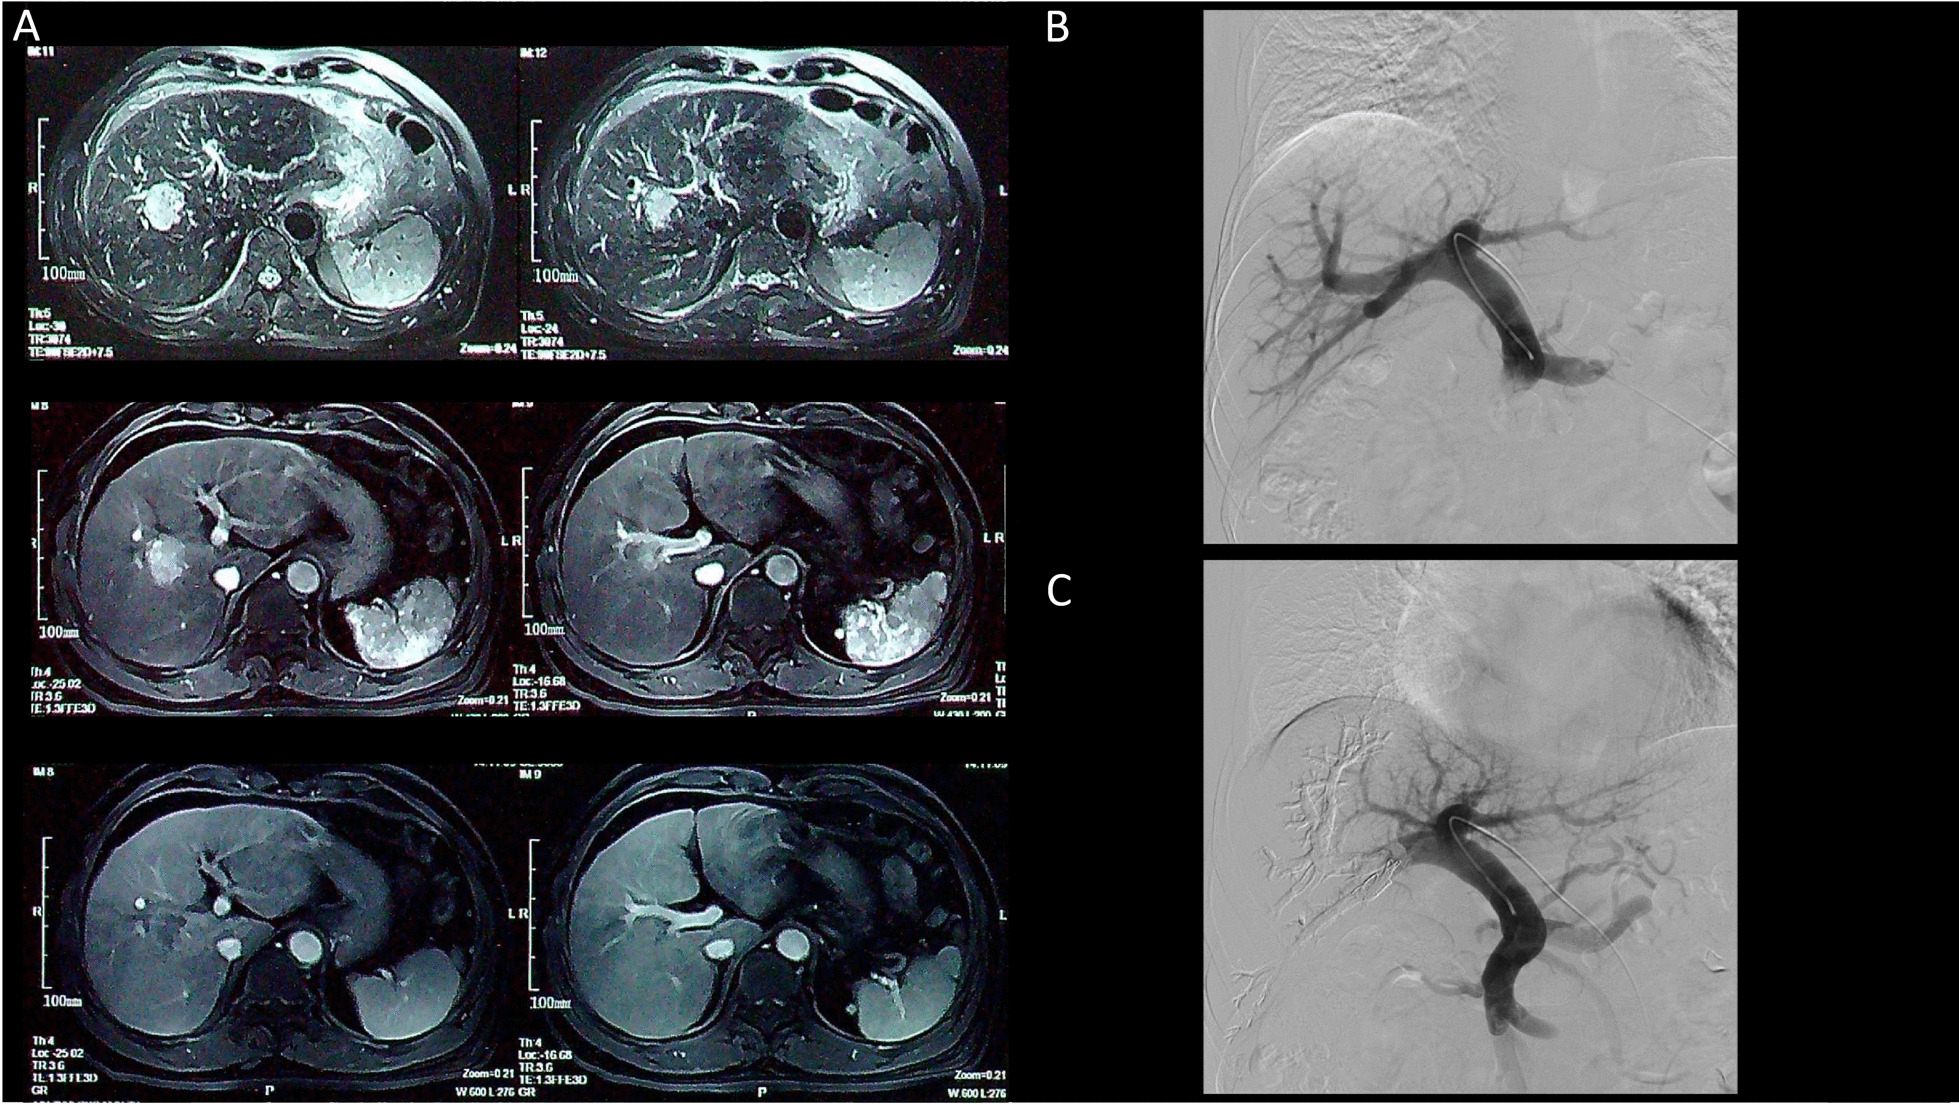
A Magnetic resonance imaging (MRI) before PVE in different phases; B Angiography of portal vein before embolism; C Angiography of portal vein after embolism
